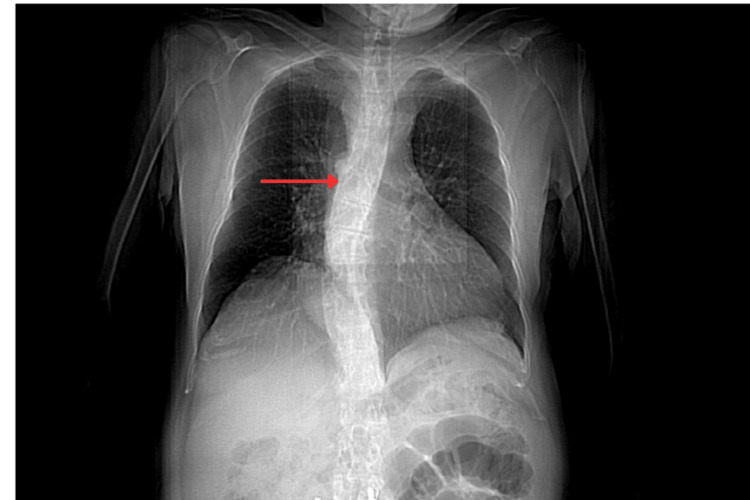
Coronal view of a CT chest showing the deviated descending aorta (red arrow)
